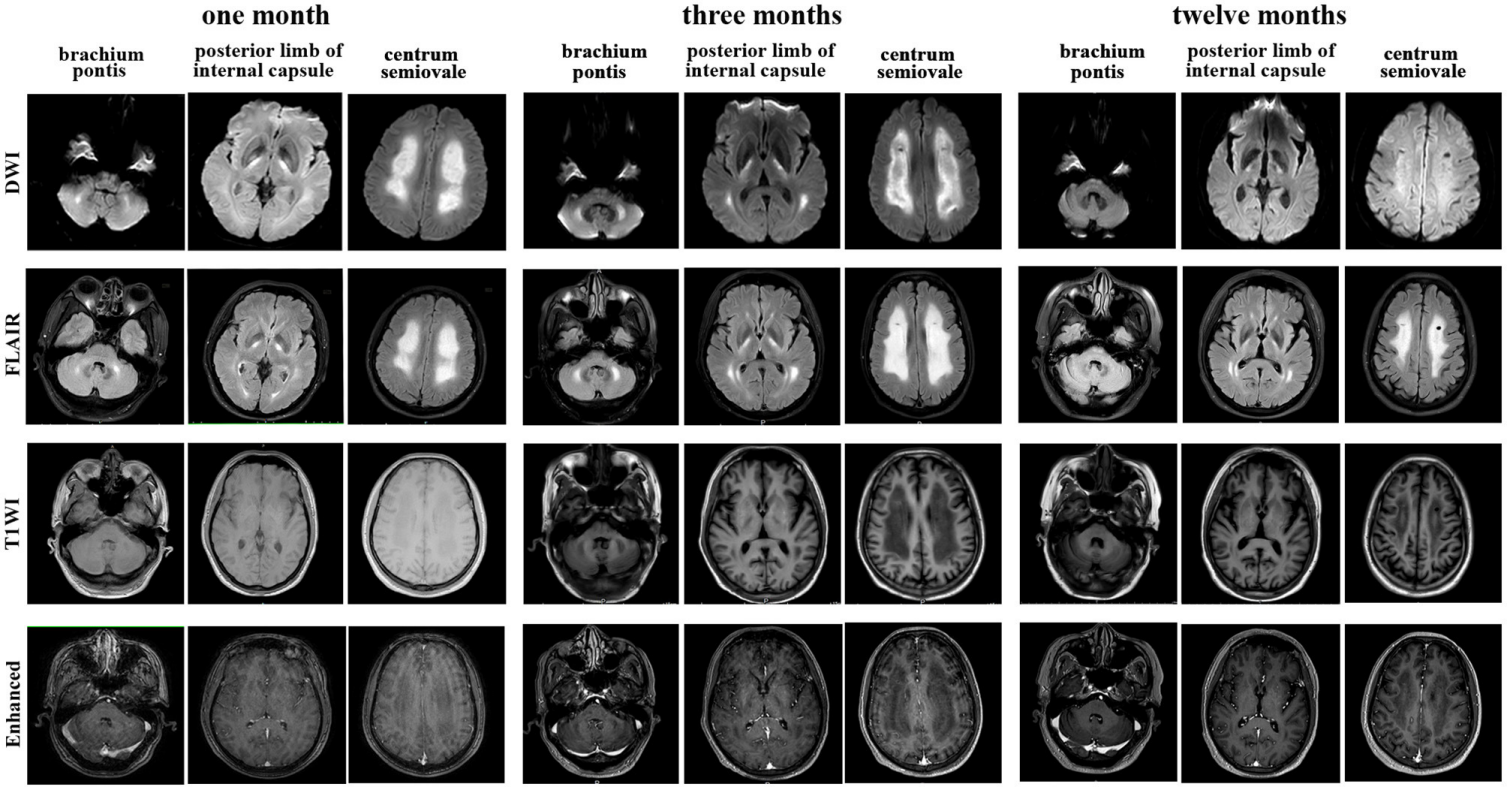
Brain magnetic resonance imaging (MRI) performed at the 1-month, 3-month, and 12-month follow-ups showed T1-weighted, diffusion-weighted images (DWI), fluid-attenuated inversion recovery (FLAIR) and enhanced T1 MRI axial images of the centrum semiovale, brachium pontis and internal capsule lesions.
